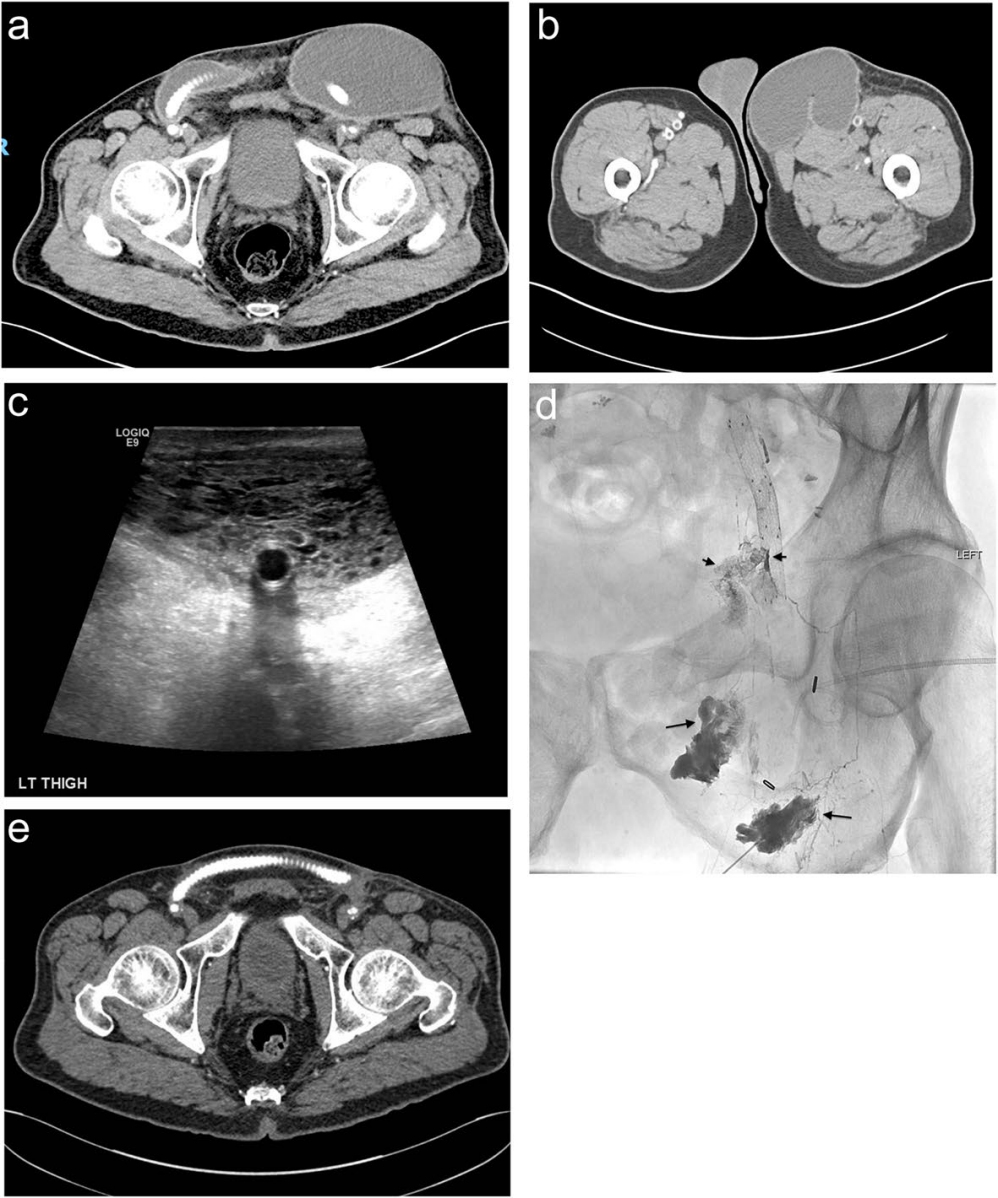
Patient with recurrent left groin lymphocele following femoral artery–femoral artery bypass graft (patient #3). a Contrast-enhanced axial CT shows a large fluid collection in the left groin extending around the femoral artery–femoral artery bypass graft. b Axial CT at a more inferior level demonstrates caudal extension of the lymphocele. c Transverse ultrasound reveals a heavily septated lymphocele within the subcutaneous soft tissues. The femoral artery stent is visible. d Lymphangiogram performed via injection into two inguinal lymph nodes shows lipiodol extravasation around the nodes (long arrows) and cranially directed lymphatic channels. There is an abrupt cutoff of a dilated lymphatic channel, with leakage into a spongiform-appearing complex lymphocele medial to the cutoff point (short arrows). A pigtail drain is seen, which was removed at the end of the procedure due to minimal output caused by extensive septations. e Follow up CT scan 40 days after the lymphangiogram demonstrates complete resolution of the complex lymphocele
